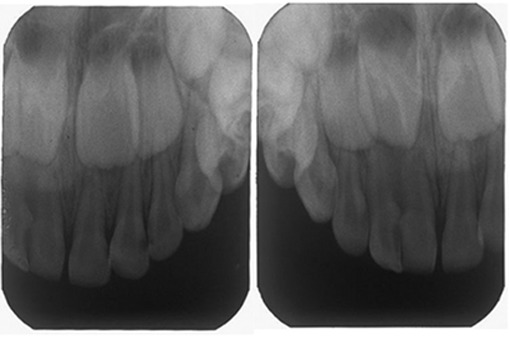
The peri-apical radiograph shows incomplete fusion of the primary maxillary left lateral incisor and the lateral supernumerary tooth. Supernumerary right lateral incisor shows a slight crown dilaceration.
